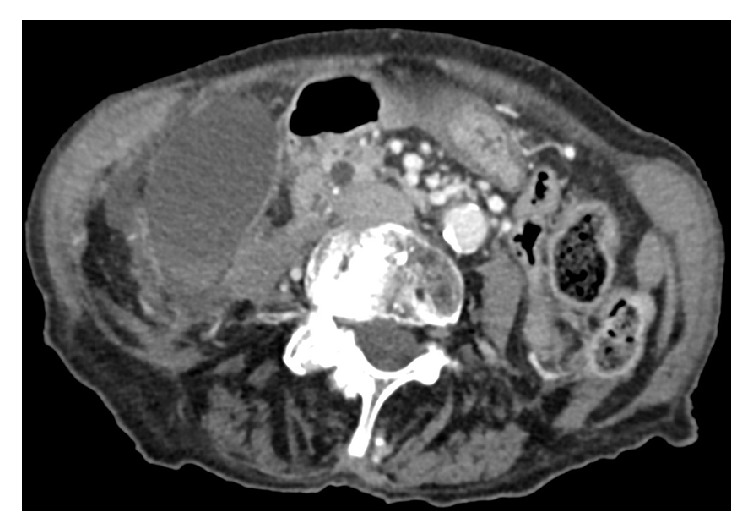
Abdominal contrast enhanced CT scan, portal phase distended gallbladder with focal enhancement defect.
